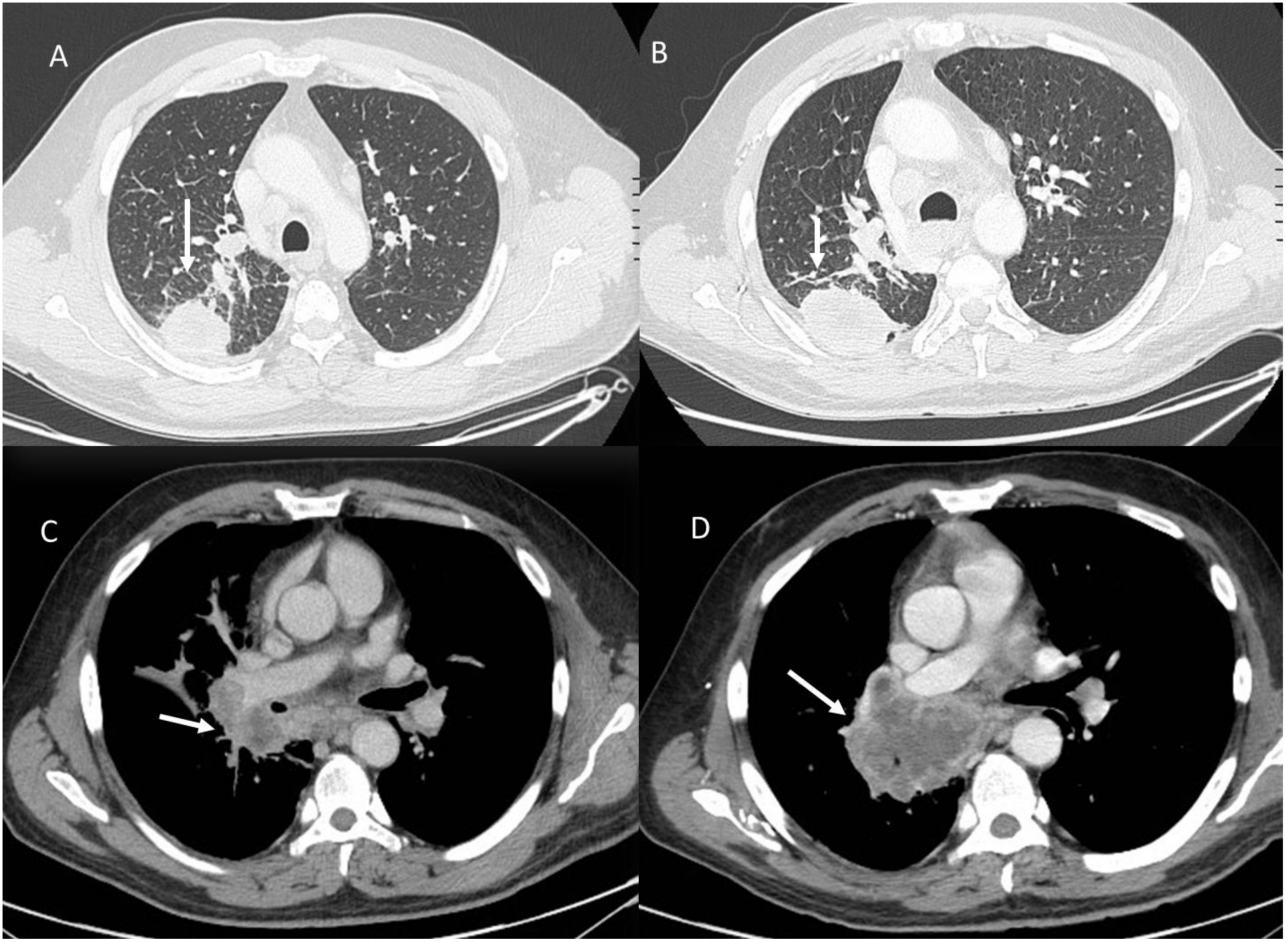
Hyperprogression during immunotherapy in a patient with lung cancer. Axial CT scans: (A) (Baseline CT with Lung Window): The initial CT scan shows a solid, spiculated mass in the left lower lobe (white arrow), with surrounding ground-glass opacities and an adjacent area of consolidation. (B) (Follow-up CT with Lung Window After Immunotherapy): Imaging performed during treatment evaluation reveals rapid tumor enlargement (white arrow) with new areas of consolidation and increased peritumoral opacities, suggesting disease progression. (C) (Baseline Contrast-Enhanced CT, Portal Venous Phase): Hilar metastasis (white arrow). (D) (Follow-up Contrast-Enhanced CT, Portal Venous Phase): Marked enlargement of the hilar metastasis (white arrow) with increased central necrosis is observed, further supporting hyperprogression. From Vanzulli and Albano, (42).
